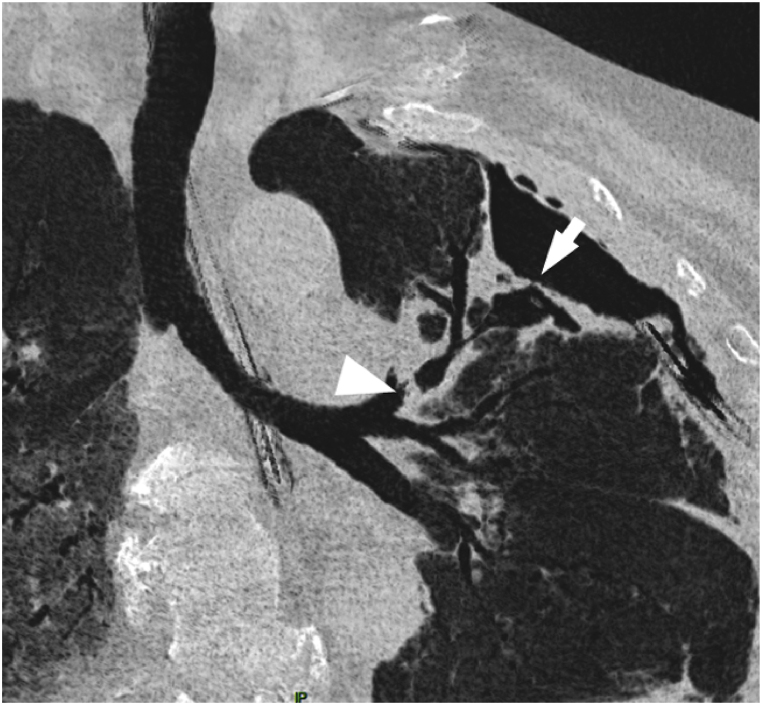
Minimum intensity projection (MinIP) on CT scan revealing alveolar-pleural fistula (White arrow), and the bronchial connection between cavitation and anterior segmental bronchus, where EBV is set (White arrow's head), in the left upper lobe.
